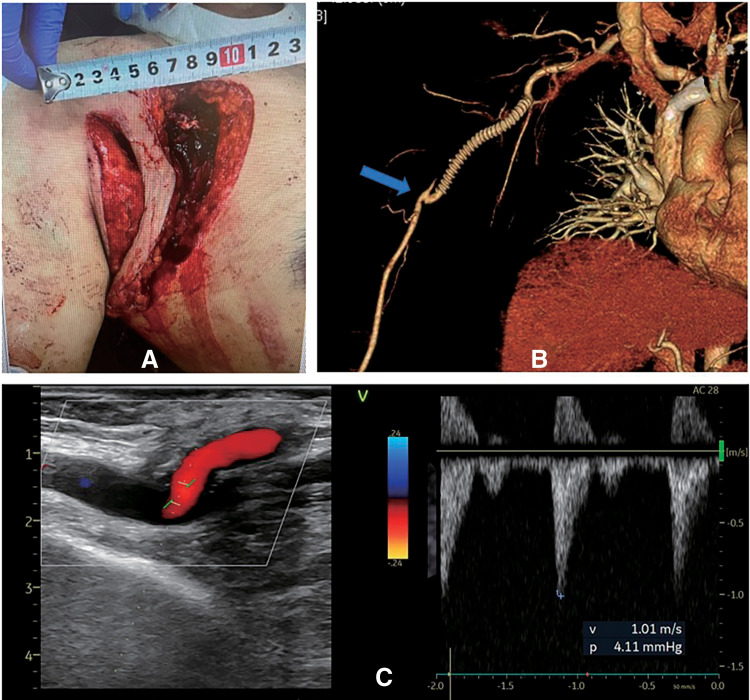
Fig. 4 A case of axillobrachial artery bypass for traumatic obstruction of the brachial artery (Patient 10). (A) A 59-year-old man fell from a height, resulting in an axillary laceration and an arteriovenous injury. Axillobrachial artery bypass was performed using a 6-mm PTFE graft. (B) 3D reconstruction of postoperatively obtained computed tomography image showing the site of the vein cuff (blue arrow). (C) Color Doppler of the vein cuff showing a patent graft, absence of significant stenosis, and high-speed turbulent flow. PTFE: polytetrafluoroethylene
